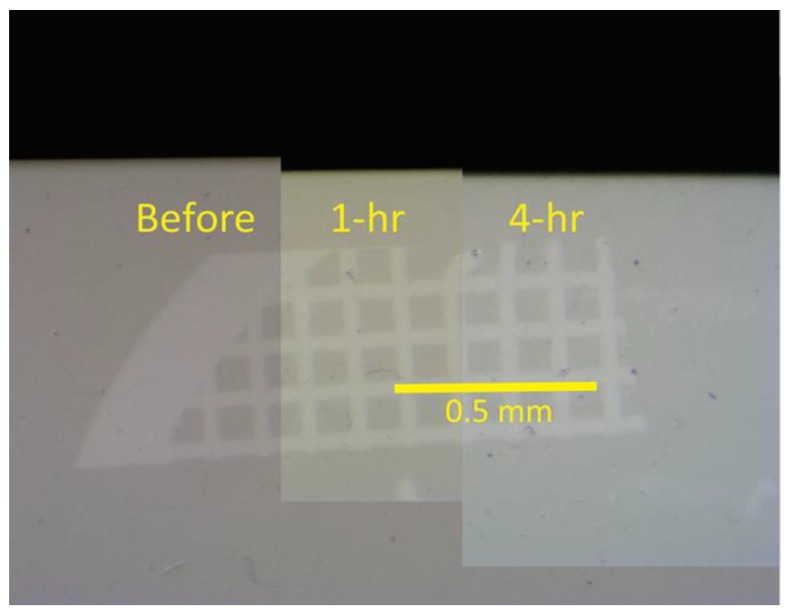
X-ray images aligned to show effect of time on citric acid erosion of dentin. Dentin sample X-rays taken before, and at 1 h and 4 h were aligned using the fiduciary TEM grid marker to assess surface mineral loss.
